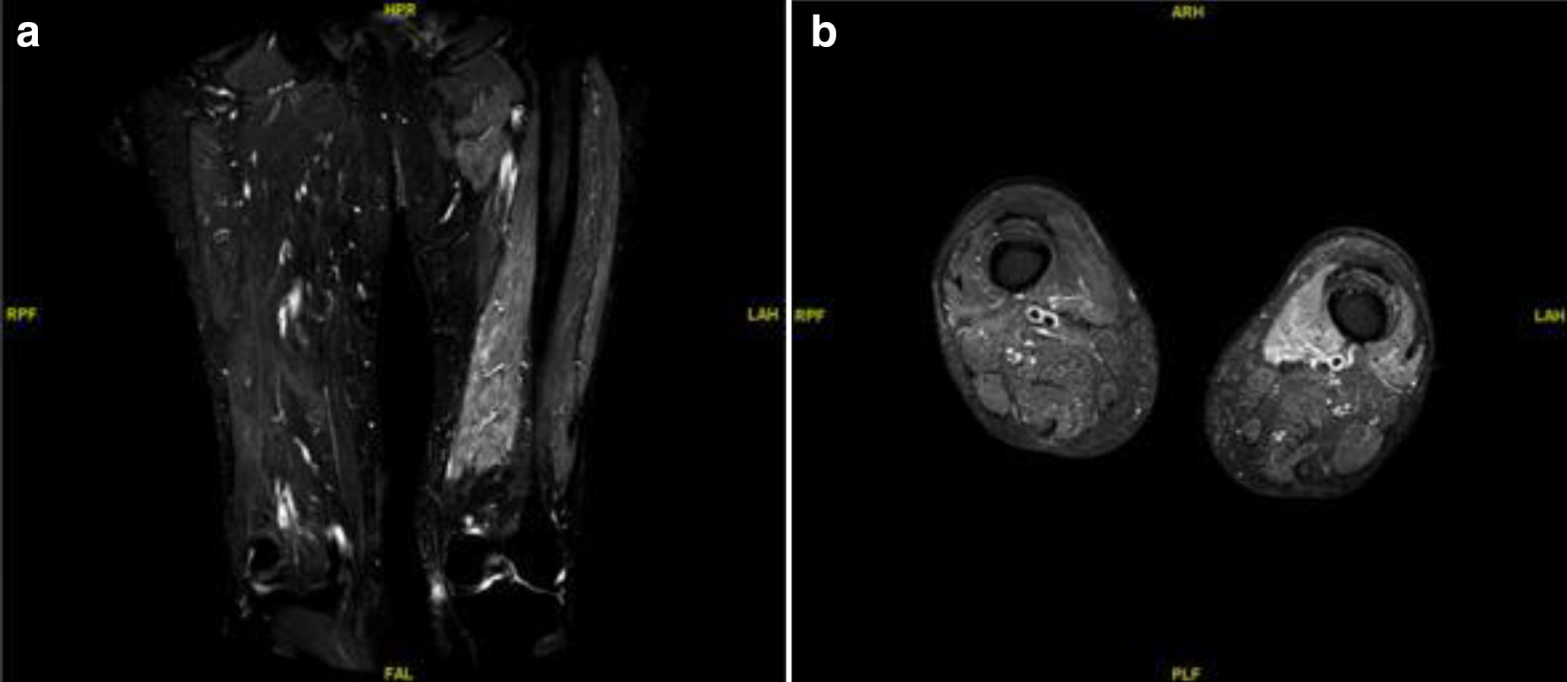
MRI of both thighs STIR sequence. (a) Coronal section STIR MRI both thighs. (b) Axial section STIR MRI both thighs. MRI of both thigh and leg reported by MSK Radiologist as marked oedema of Vastus Medialis, Adductor compartment, and even going to Tibialis Anterior of left side. Myositis of unknown aetiology suggested. Potentially due to inflammatory or ischemia process. Infectious aetiology is possible but less likely. STIR, short tau inversion recovery
